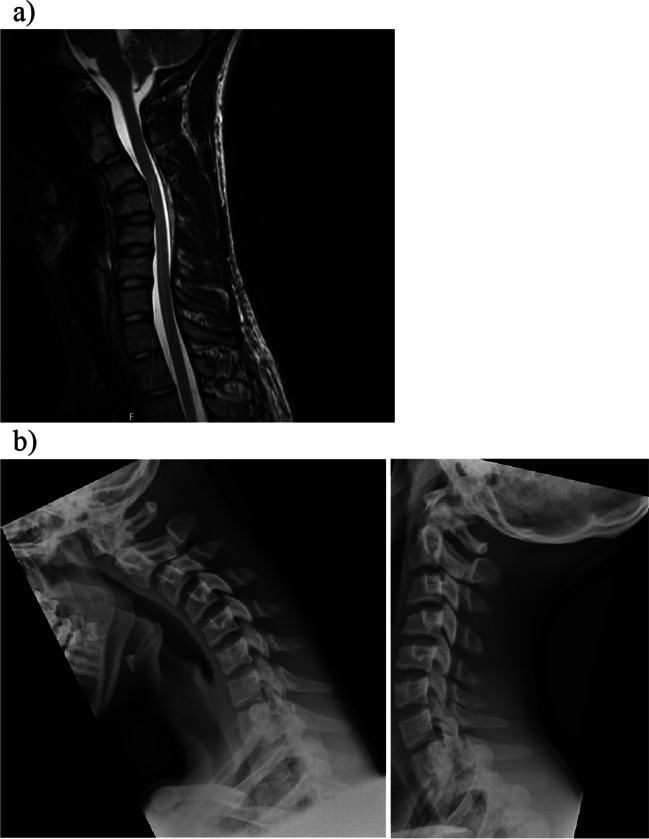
a Pre-operative sagittal non flexion T2-weighted MRI of Hirayama’s disease with kyphosis at C2-C5 and anterior dural shifting with epidural flow-voids. b Pre-operative flexion and extension x-ray revealed a kyphotic deformity between segments C3 and C5 that partially reduced in hyperextension
